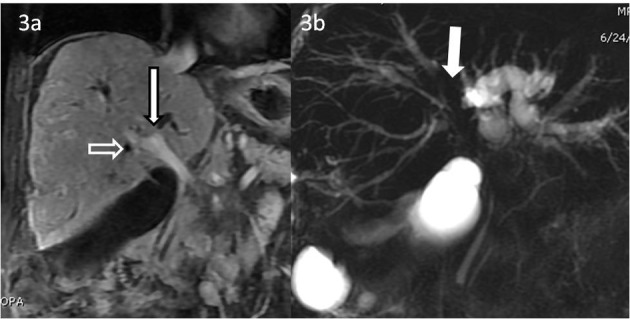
CEMRI T1-weighted fat suppressed sequence performed with gadolinium BOPTA showing lesion extent on the coronal sequences. (a) Coronal image of the dynamic CEMRI T1-weighted fat suppressed sequence performed with Gd BOPTA in the equilibrium phase showing lesion extension to the porta hepatis (bold white arrow with black margins) and encasement of the right portal vein (white outlined arrow). (b) Coronal 3D MRCP shows non-visualized primary biliary confluence with resultant moderate left lobe and mild right lobar ductal dilatation (bold white arrow pointing at the confluence).
